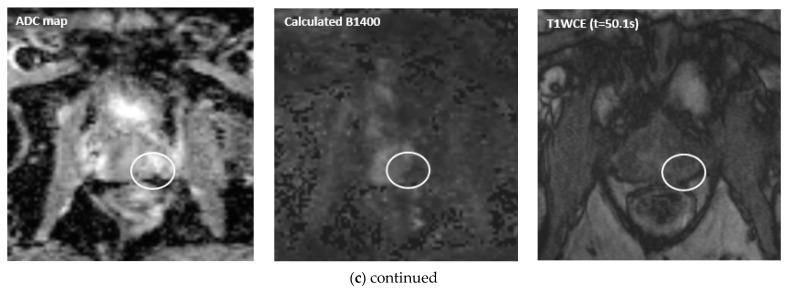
Recurrent PCa in a 72-year-old patient with a PSA level of 8.0 ng/mL (Gleason score 3 + 4 = 7) after initial treatment with external beam radiation therapy. (a) Pre-treatment multiparametric MR images: Axial T2W image, ADC map, high *b* value and contrast-enhanced image, respectively. Tumor is located in the left peripheral zone. The maximum lesion dimensions in anterior-posterior (AP), left-right (LR) and craniocaudal (CC) are 22, 10 and 20 mm. (b) Axial T1W MR image demonstrating prostate cryoablation with 3 cryoneedles (Ice-Seeds) positioned under real-time guidance. Axial image with the iceball sharply visualized as a signal void area surrounded by a hyperintense rim corresponding to 0 °C (arrowhead). The urethra (*) and rectum (arrow) contain an endoluminal urethral warming catheter and rectal warming balloon to protect the urethra and rectum from freezing, respectively. (c) Post-treatment multiparametric MR images at one year follow-up (PSA level of 2.2 ng/mL): Axial T2W image demonstrates the ablation zone with tissue changes. Axial ADC map and high *b* value image show no restricted diffusion. Axial dynamic contrast-enhanced MR image demonstrates a slight enhancement in the treated area. MRI-guided targeted biopsies showed a focus of adenocarcinoma with a maximum length of 1 mm. The patient continued on active surveillance.
